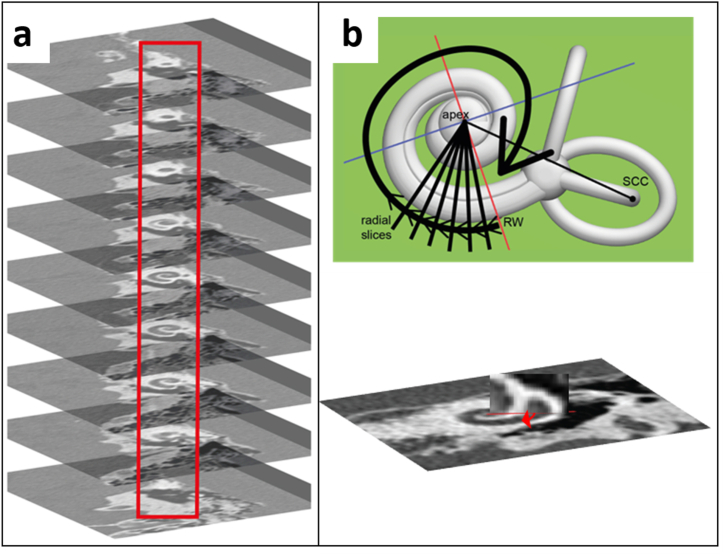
Re-slicing of the CT dataset. a, Slices were parallel to the basal turn of the cochlea. b, Radial mid-modiolar re-slicing with the modiolus as the central axis, starting at the round window (RW) and perpendicular to the planar slices.
